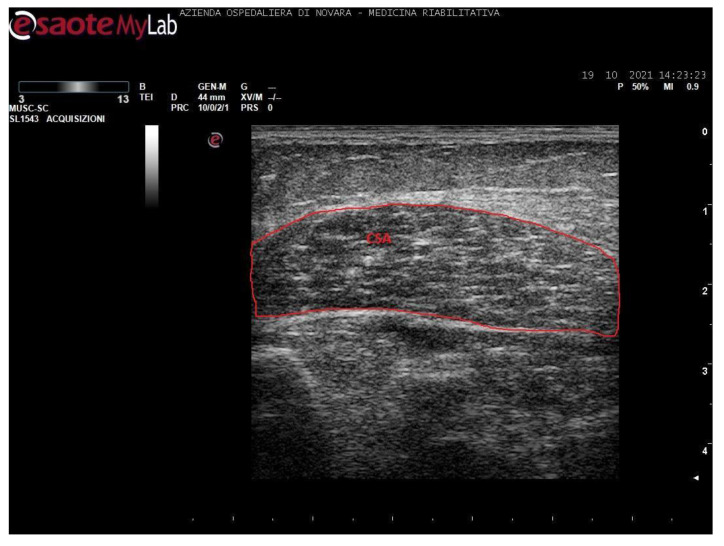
Ultrasound image of soleus in transversal section. In red: cross-sectional area (CSA).
